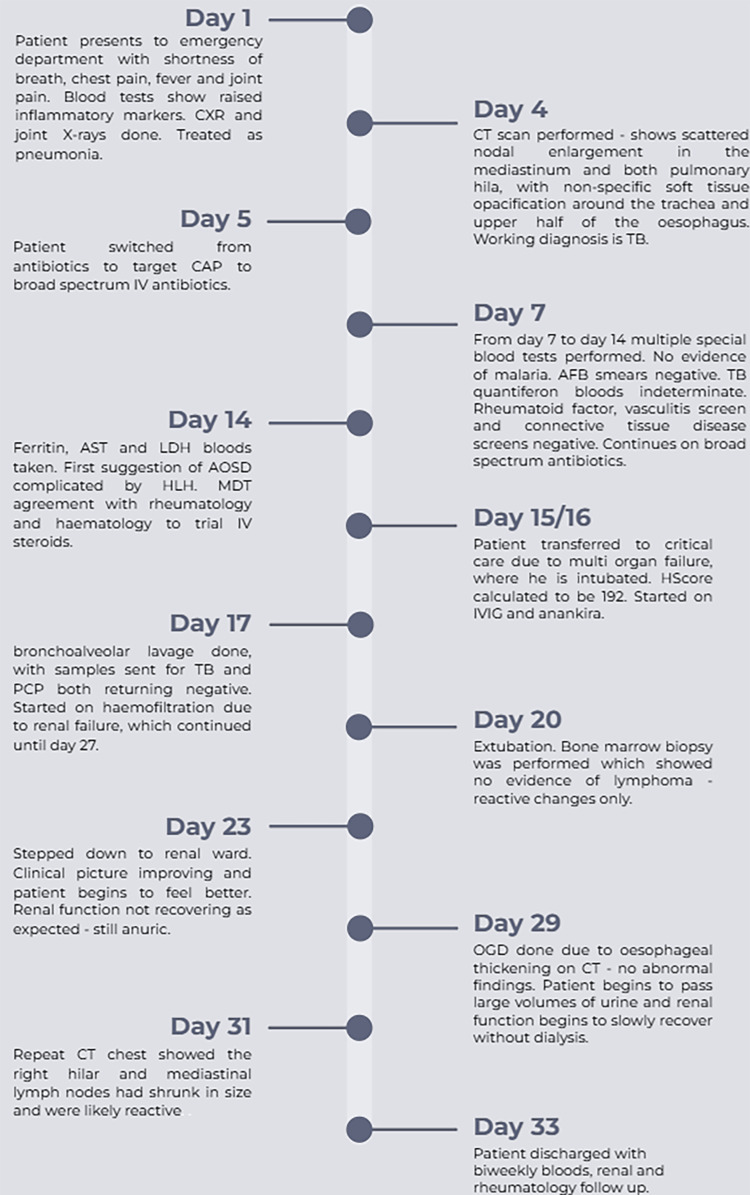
Timeline showing events of admission CXR: chest X-ray, CT: computed tomography, TB: tuberculosis, CAP: community-acquired pneumonia, AFB: acid-fast bacilli, AOSD: adult-onset Still's disease, HLH: haemophagocytic lymphohistiocytosis, MDT: multidisciplinary team, IV: intravenous, H-score: hemophagocytic syndrome diagnostic score, IVIG: intravenous immunoglobulin, PCP: Pneumocystis pneumonia, OGD: oesophago-gastro-duodenoscopy, AST: aspartate aminotransferase, LDH: lactate dehydrogenase
